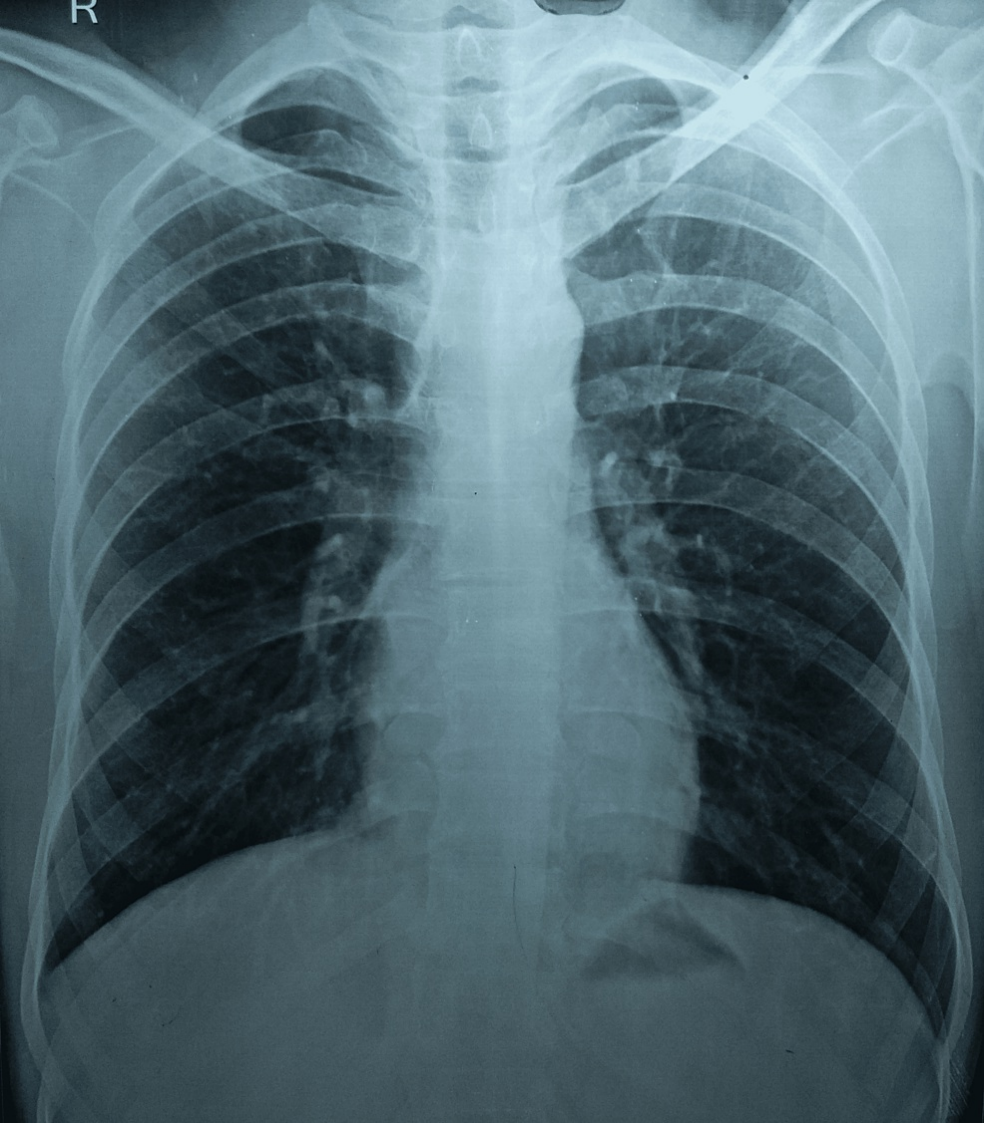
A normal chest radiograph (P-A view) P-A, Postero-anterior
